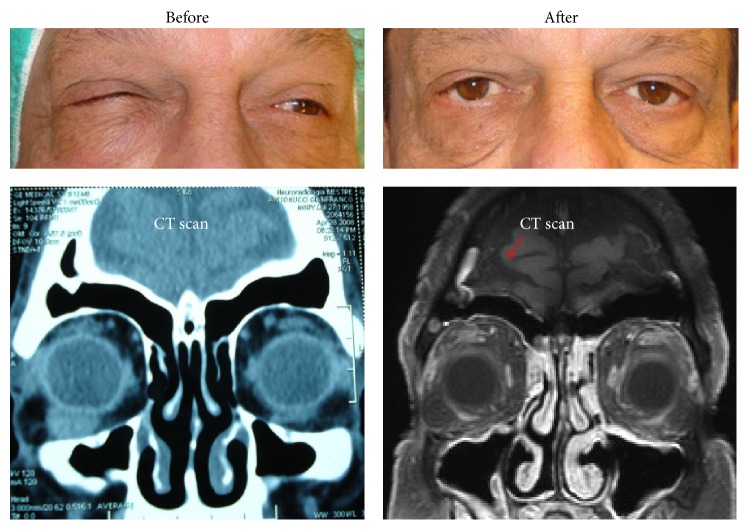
GCT before and after surgery. Top panel shows proptosis before (left) and after (right) surgery. Bottom panel shows CT scans to identify the GCT (left), and to confirm the absence of the mass after surgery (right).
